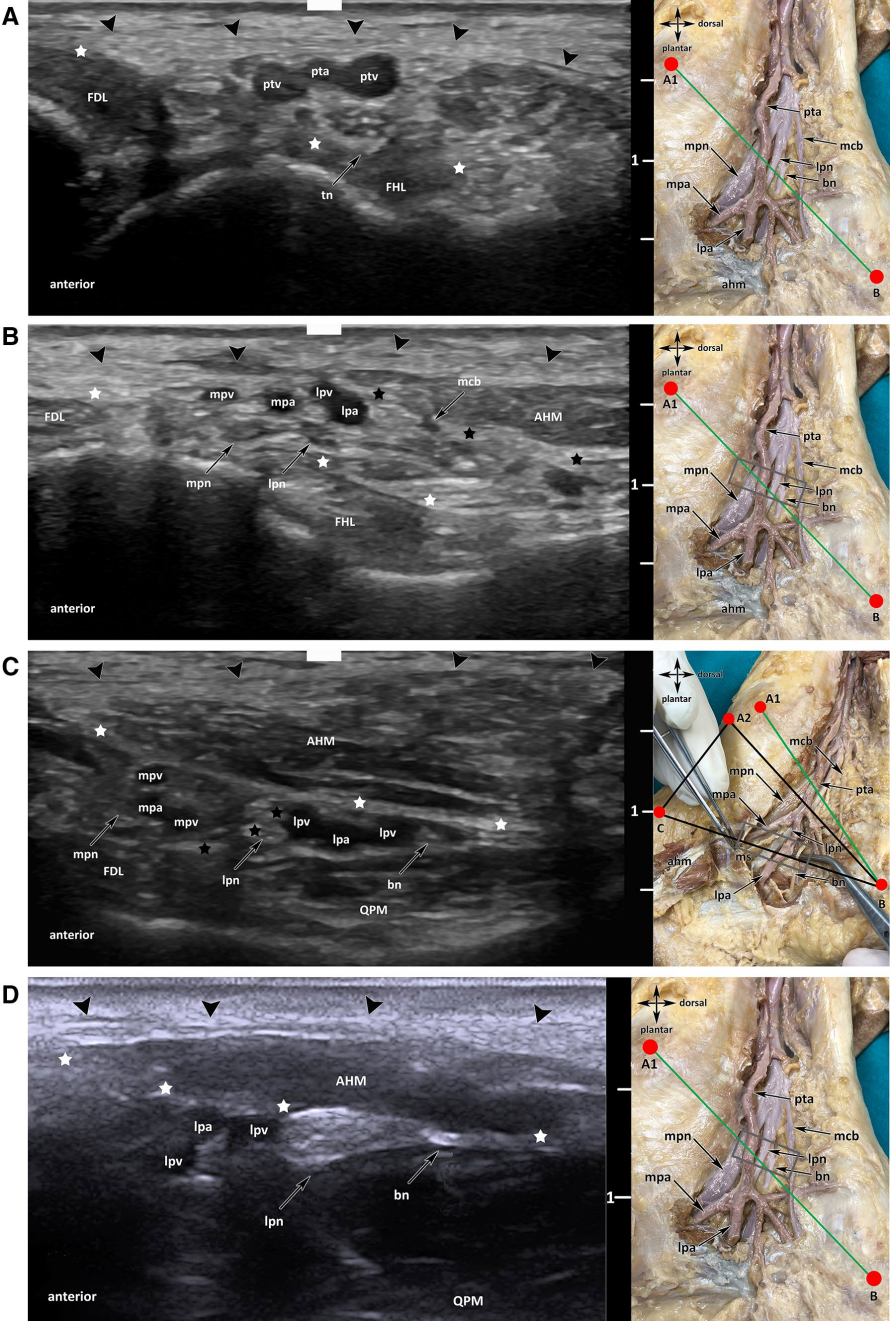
a Ultrasound visualization of TN. *tn* tibial nerve, *FDL* flexor digitorum longus, *FHL* flexor hallucis longus, *pta* posterior tibial artery, *ptv* posterior tibial veins, black arrowheads: superficial layer flexor retinaculum, white stars: profound layer flexor retinaculum. b Ultrasound visualization of the tibial division and the medial calcaneal branch. *Mpn* medial plantar nerve, *lpn* lateral plantar nerve, *mcb* medial calcaneal branch, *FDL* flexor digitorum longus, *FHL* flexor hallucis longus, *AHM* abductor hallucis muscle, *lpa* lateral plantar artery, *lpv* lateral plantar vein, *mpa* medial plantar artery, *mpv* medial plantar vein, thick arrows:superficial layer flexor retinaculum, white stars:profound layer flexor retinaculum, black stars:medial septum. c Ultrasound visualization of the terminal branches (lpn, mpn, bn) on [BC] line. *Lpn* lateral plantar nerve, *mpn* medial plantar nerve, *bn* Baxter’s nerve, *FHL* flexor hallucis longus muscle, *QPM* quadratus plantae muscle, *AHM* abductor hallucis muscle, *mpv* medial plantar vein, *lpv* lateral plantar vein, *mpa* medial plantar artery, *lpa* lateral plantar artery, black arrowheads: superficial layer flexor retinaculum, white stars: medial septum (deep fascia of AHM), black stars: medial septum (interfascicular septum). d Ultrasound visualization of the arising of BN on [A_1_B] line. *Lpn* lateral plantar nerve, *bn* Baxter’s nerve, *lpv* lateral plantar vein, *lpa* lateral plantar artery, *AHM* abductor hallucis muscle, *QPM* quadratus plantae muscle, black arrowheads: superficial flexor retinaculum, white star: profound layer flexor retinaculum
